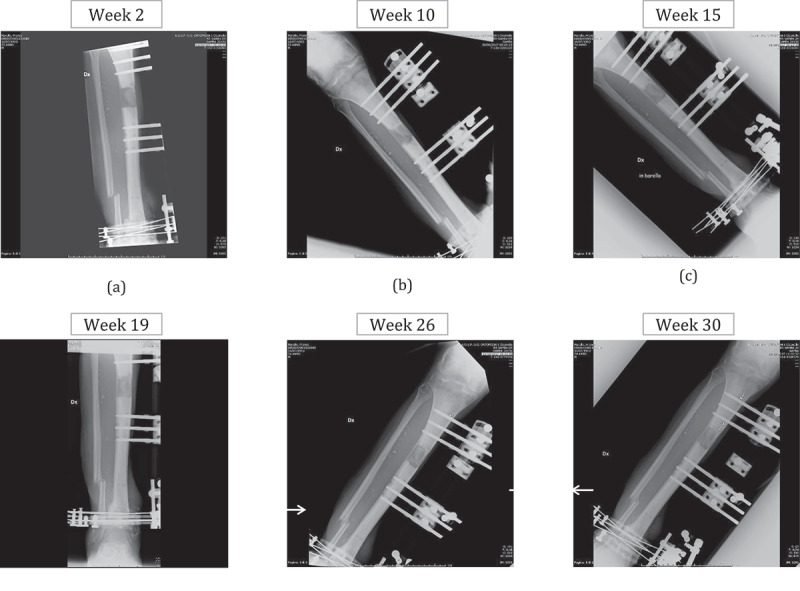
X-rays during the fracture healing
